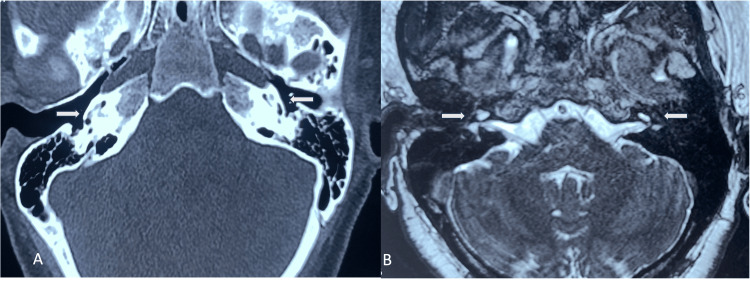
Radiology of the temporal bone showing ossification of the cochlea A: High-resolution CT of temporal bone showing the presence of partial ossification (white arrow) involving the basal turn of cochlea on the right side and both basal and middle turn on the left side. B: T2 weighted image of MRI scan showing faint fluid signal (white arrow) in the basal turn of left cochlea and middle and apical turns on the right side
